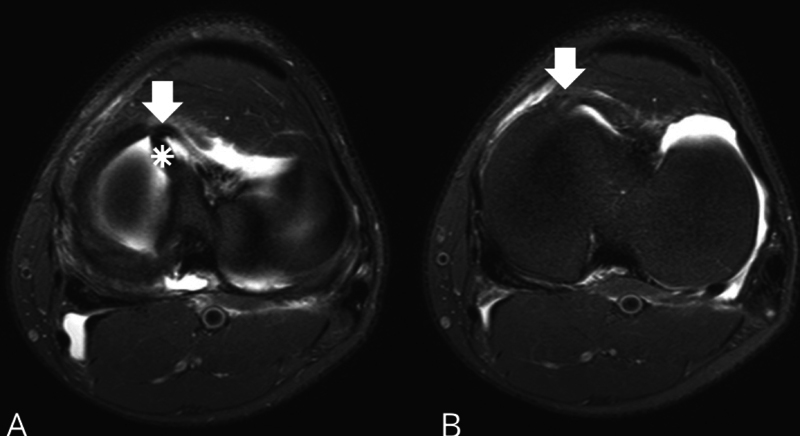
Cross-sections of a preoperative MRI scan of a 27 year-old male patient with a bucket-handle tear of the medial meniscus of the left knee. (
